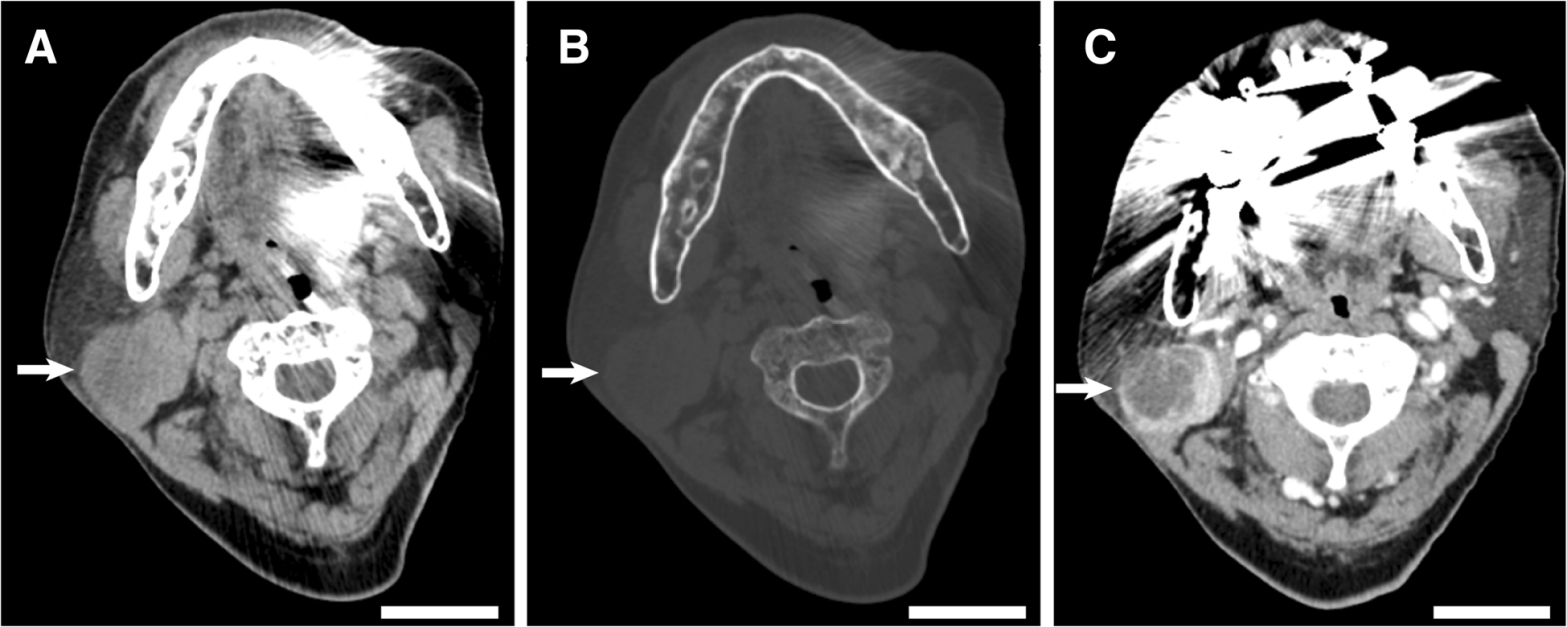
Radiographic images of the tumor. a–b Axial non-enhanced computed tomography images in soft tissue window (a) and bone window (b) show a nodular lesion (arrow) in the right parotid gland. c Contrast-enhanced computed tomography image shows the cystic and solid appearance of the tumor (arrow). *Bars* represent 5 cm
